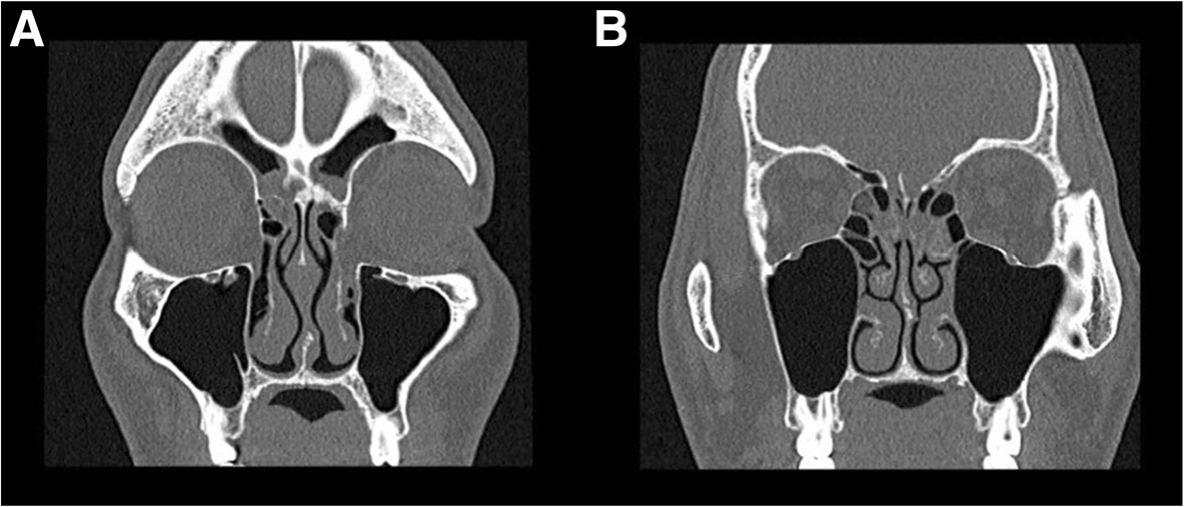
a - Radiographic evidence of CCAD with central disease and peripheral clearing; b - “black halo”sign
